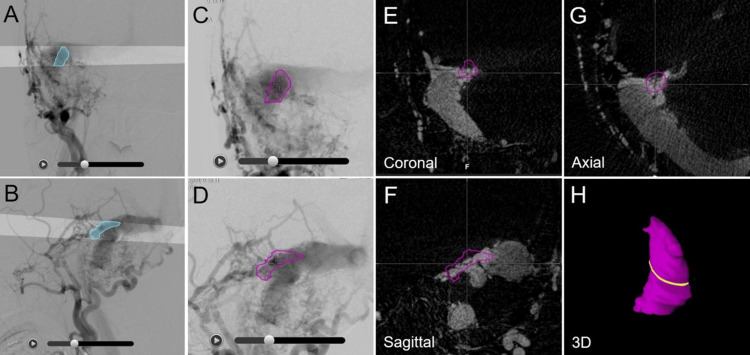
Target contouring process after 2D/3D angiogram fusion for TS-DVAF. A–D: 2D-DSA (A, C: frontal view; B, D: lateral view); E–G: CBCTA subtraction images; H: 3D view of an automatically generated 3D object (purple) based on the tentative outlining of the target on the DSA pair. A, B: Tentative 2D outlining of the dominant arteriovenous shunt portion (blue) on the 2D-DSA pair. Each highlighted region of interest projects a ray onto the other view and intersects appropriately. The user can review the entire frame of the 2D-DSA pair by scrolling through the images using sliders. This 3D structure (purple) leaves room for further modification to generate an appropriate target structure without over- or under-coverage.
